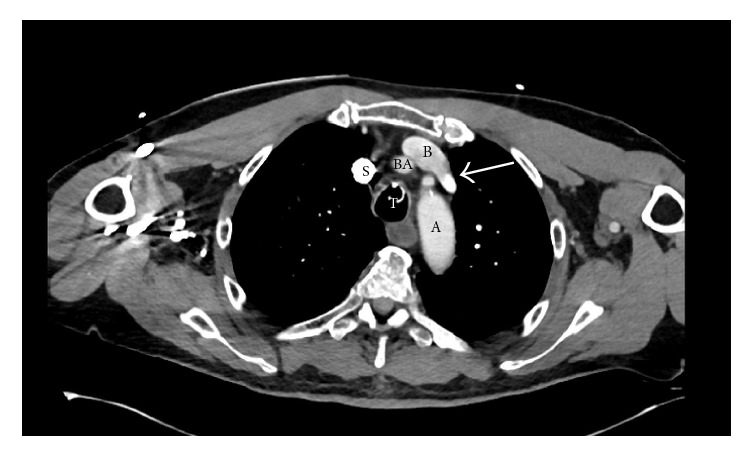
Cross section CTA of chest. S = superior vena cave, B = innominate vein, BA = innominate artery, T = trachea, and A = aorta. Arrow points to the insertion of aberrant left superior pulmonary vein into innominate vein.
